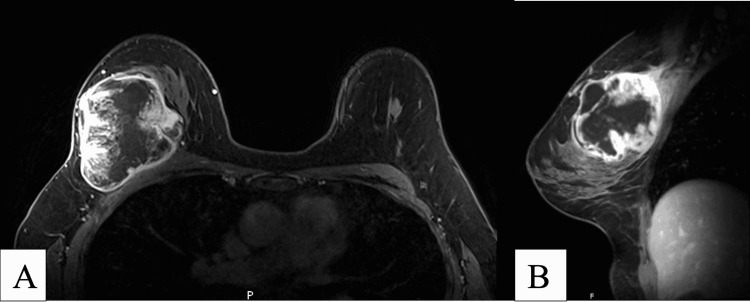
MRI performed prior to radiation therapy (A) Axial T1 MRI demonstrating heterogenous enhancement in the mass in the central outer aspect of the right breast with central necrosis; (B) Sagittal MRI images demonstrating large mass with heterogenous enhancement
